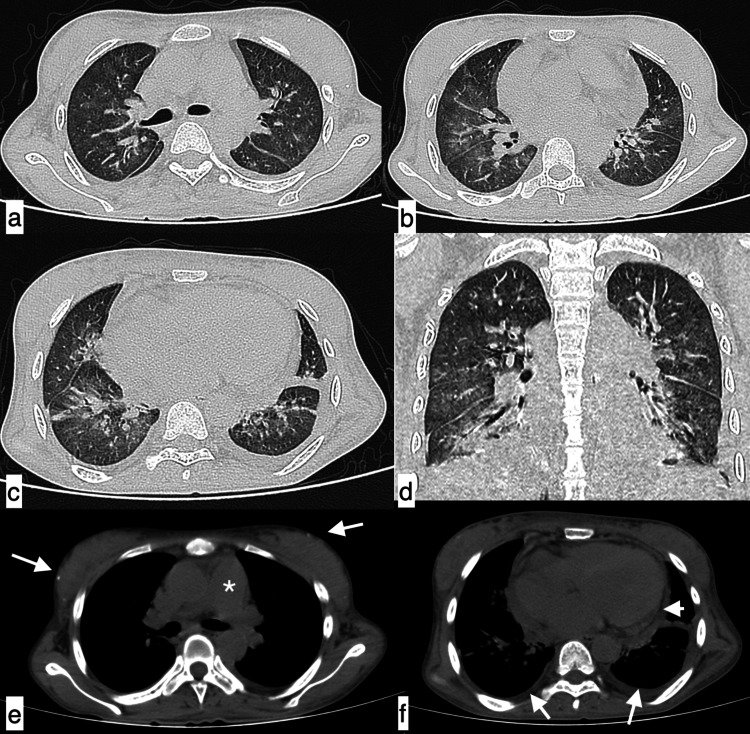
Diffuse alveolar haemorrhage A 27-year-old female patient with SLE presented with complaints of shortness of breath, fever, chest pain, and cough of four-day duration. Axial (a-c) and coronal (d) HRCT chest images in the lung window revealed symmetrical ground-glass opacities in both central and peripheral lung fields. Additionally, in the mediastinal window (e, f), an enlarged pulmonary artery (asterisk), mild pericardial (f, arrowhead) and bilateral pleural effusions (f, arrows), and cardiomegaly were also noted. Incidentally, vascular calcifications were observed in both breasts (e, arrows). Findings were consistent with diffuse alveolar haemorrhage. SLE: systemic lupus erythematosus; HRCT: high-resolution CT
